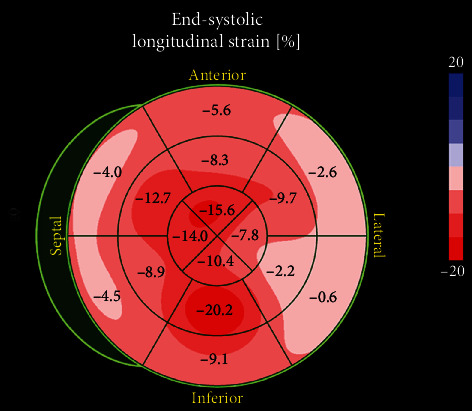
Echocardiographic strain analysis showing a relative sparing of the apex compared to the bases.
